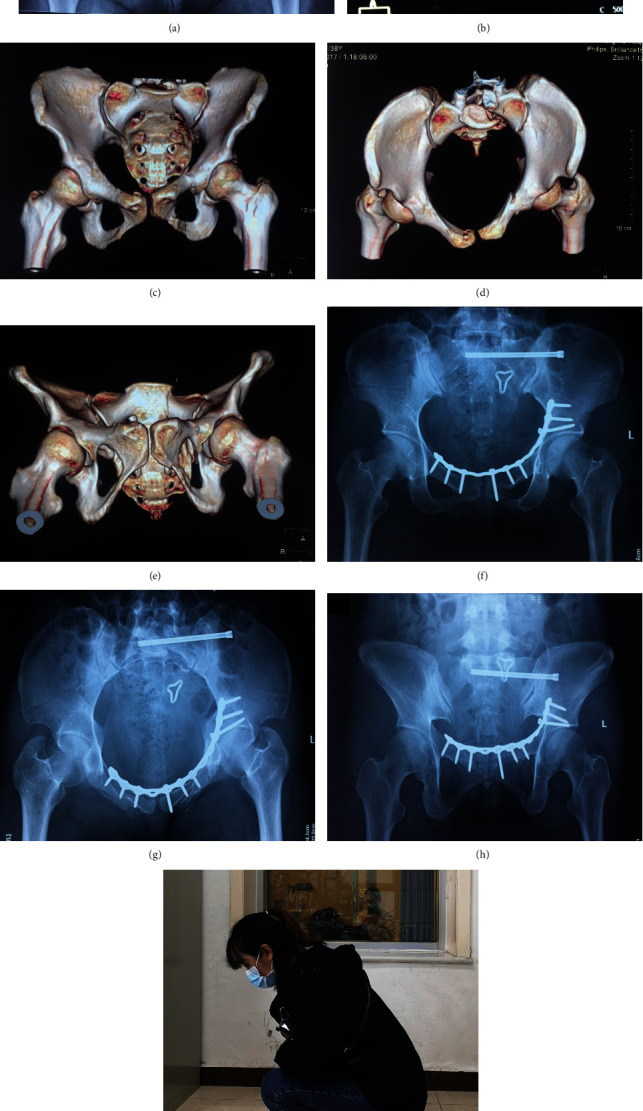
Radiographs of 32-year-old female with pelvic ring fractures (Young and Burgess LC-1/Tile B2). Preoperative AP view (a), CT scan (b), and 3-D reconstruction (c–e) showed left pubic rami and ipsilateral transforaminal sacral fracture with significant displacement. The pubic rami fracture was reduced and fixed through modified Stoppa approach followed by a sacroiliac screw inserted percutaneously. Postoperative radiographs (f–h) showed the reduction and fixation was well. The functional photograph (i) showed recovered well.
